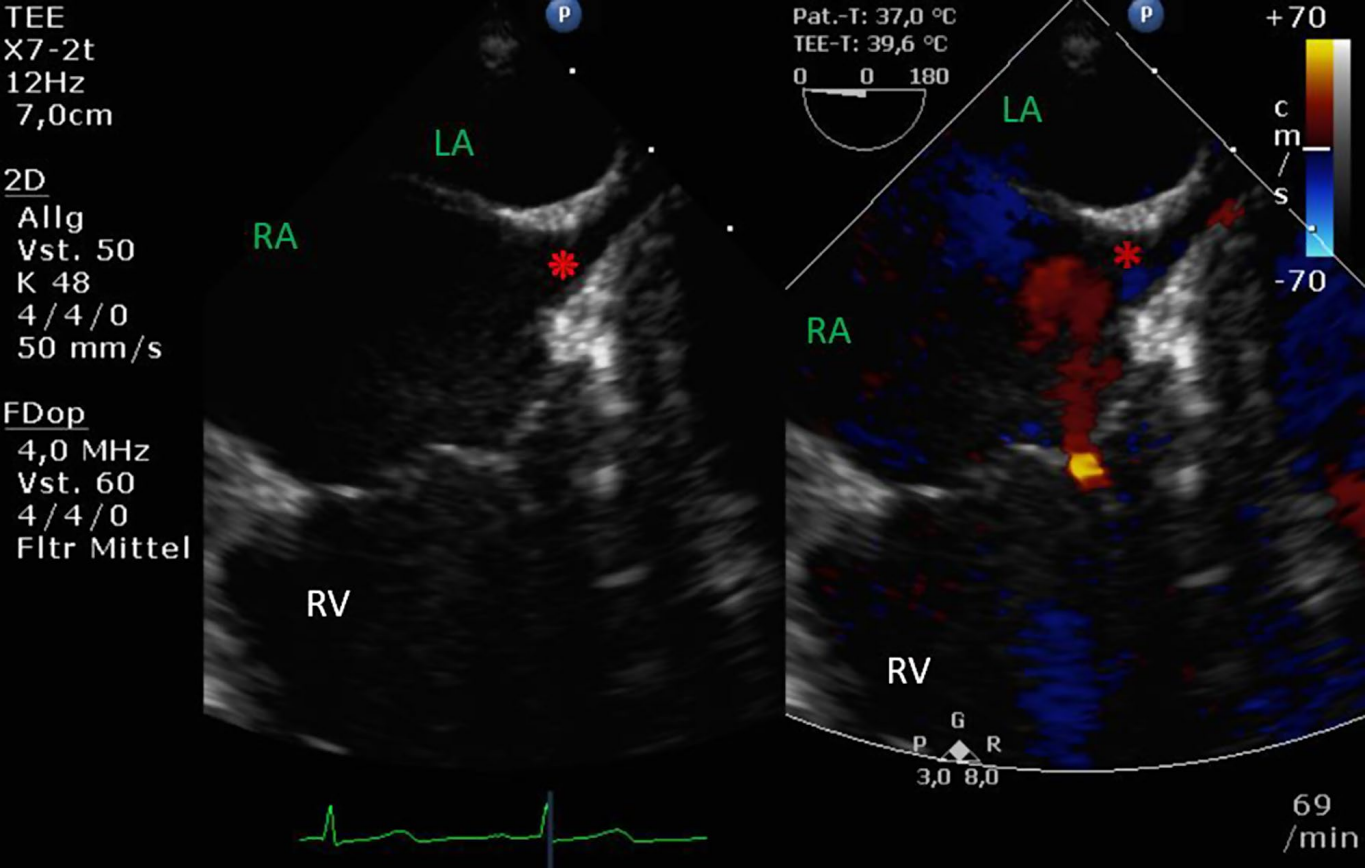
Perioperative comprehensive transesophageal echocardiographic exam of Patient B showing a suspected unroofed coronary sinus (UCS), mid esophageal two chamber view at 0°, focused to the right heart. LA: left atrium; RA: right atrium; RV: right ventricle; red circle: air bubbles; *: suspected unroofed coronary sinus
